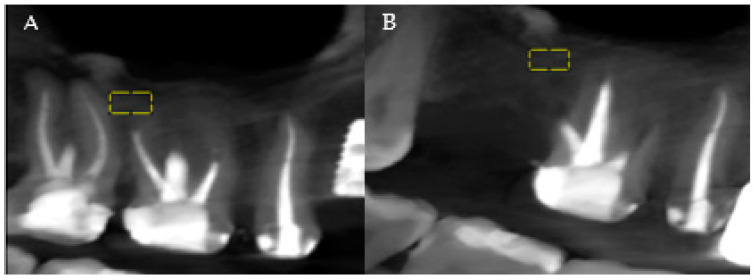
(A) Maxillary CBCT—before extraction. (B) Maxillary CBCT—after extraction. Yellow = areas of interest.
